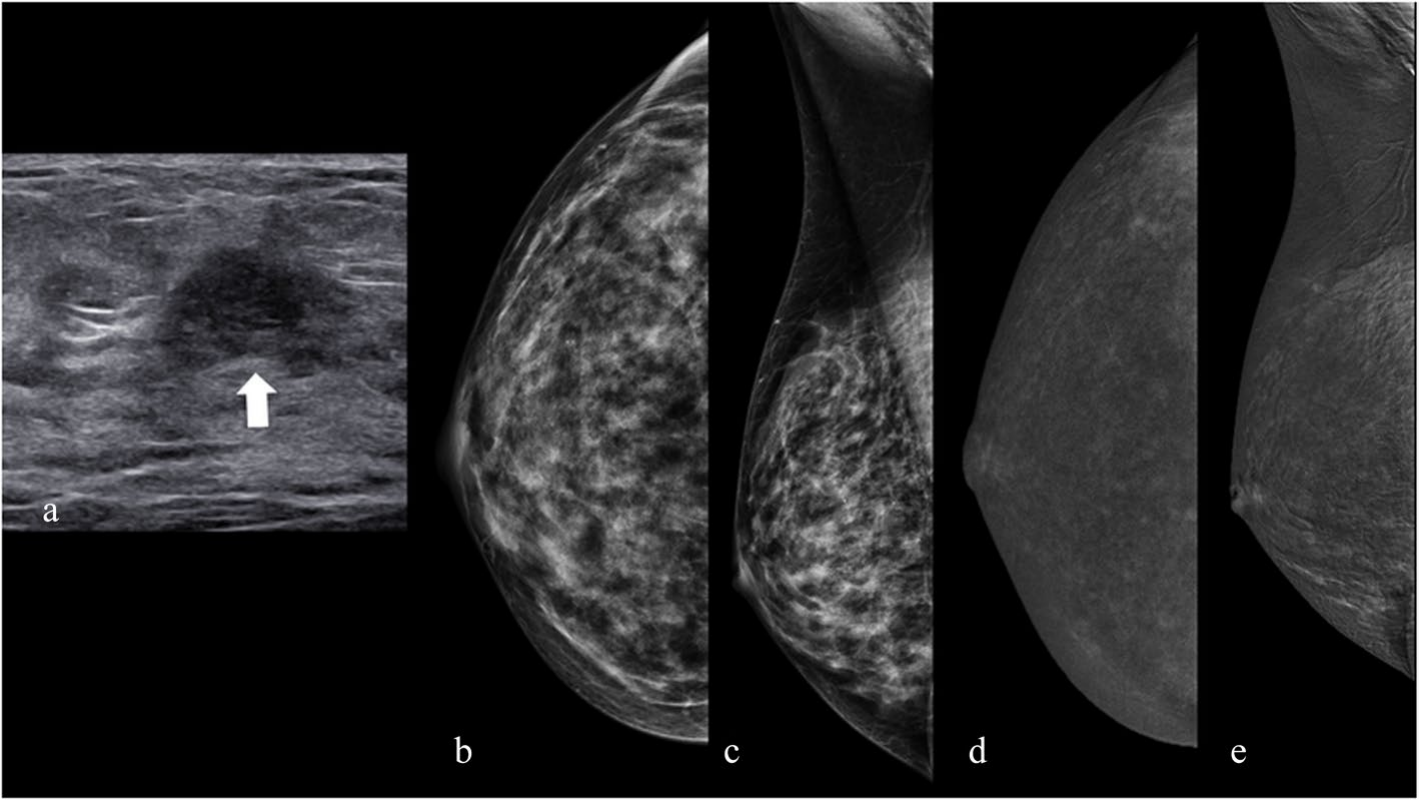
The absence of any suspicious findings is noted in both the RC and LE images corresponding to the location in the breast where a nodule was detected. The nodule presented challenges in interpretation through breast ultrasound
